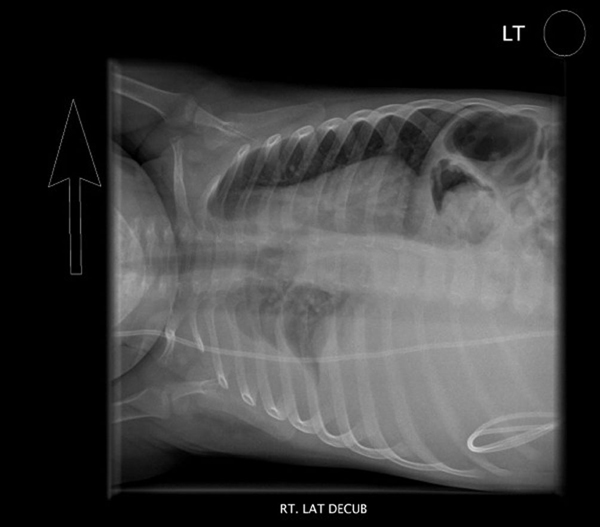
Right lateral decubitus radiograph demonstrating a moderate to large right-sided pleural fluid collection which is predominantly subpulmonic in location.
